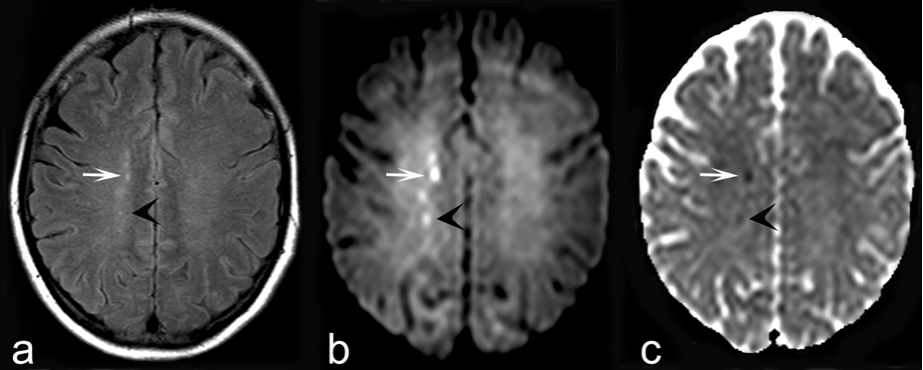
The axial FLAIR image (1A) shows small hyperintense foci in the watershed area in the right hemisphere. Some of these foci show high signal intensity on DWI (1B) and low signal intensity at the ADC-map (1C), consistent with restrictive diffusion (arrows).
